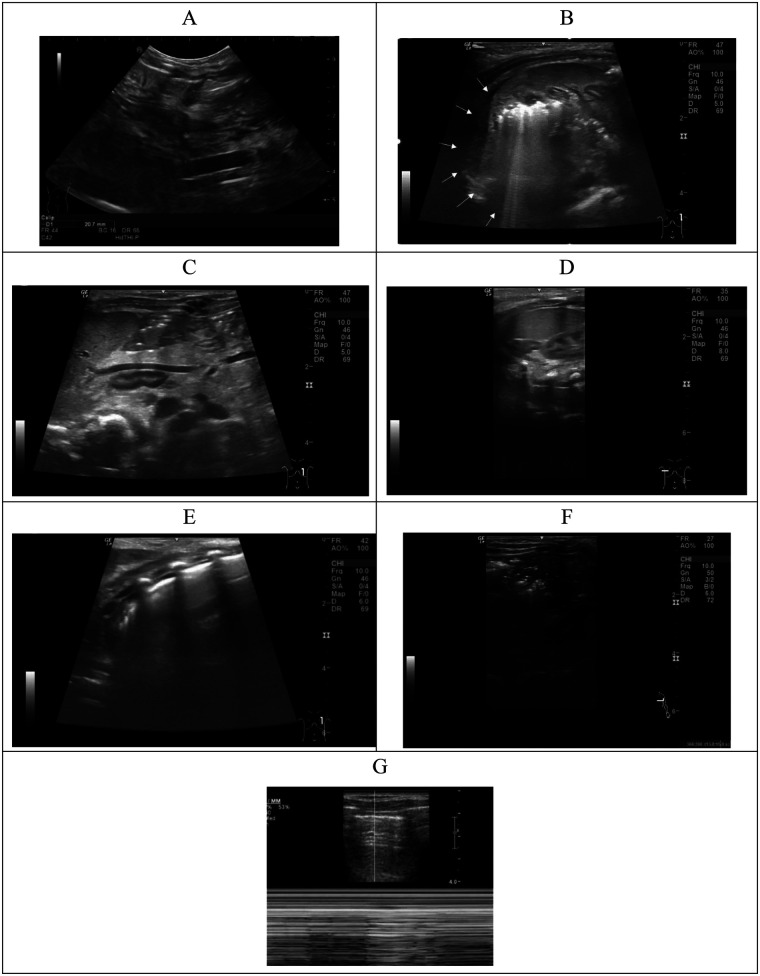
Ultrasonic diagnosis in children with CDH. (A) Measurement of diaphragmatic defect length (calipers); (B) presence of a hernia sac (arrowhead); (C) herniated abdominal contents, including the spleen, left adrenal gland, stomach, and intestinal loops, without liver involvement (infra-hepatic type); (D) herniated contents involving partial liver (L) protrusion into the thoracic cavity (suprahepatic type); (E) lung ultrasound showing interstitial edema, characterized by multiple, confluent B-lines; (F) atelectasis with the presence of an air bronchogram sign; (G) pneumothorax demonstrated on M-mode ultrasound by the stratosphere sign (parallel lines) and the absence of lung sliding.
